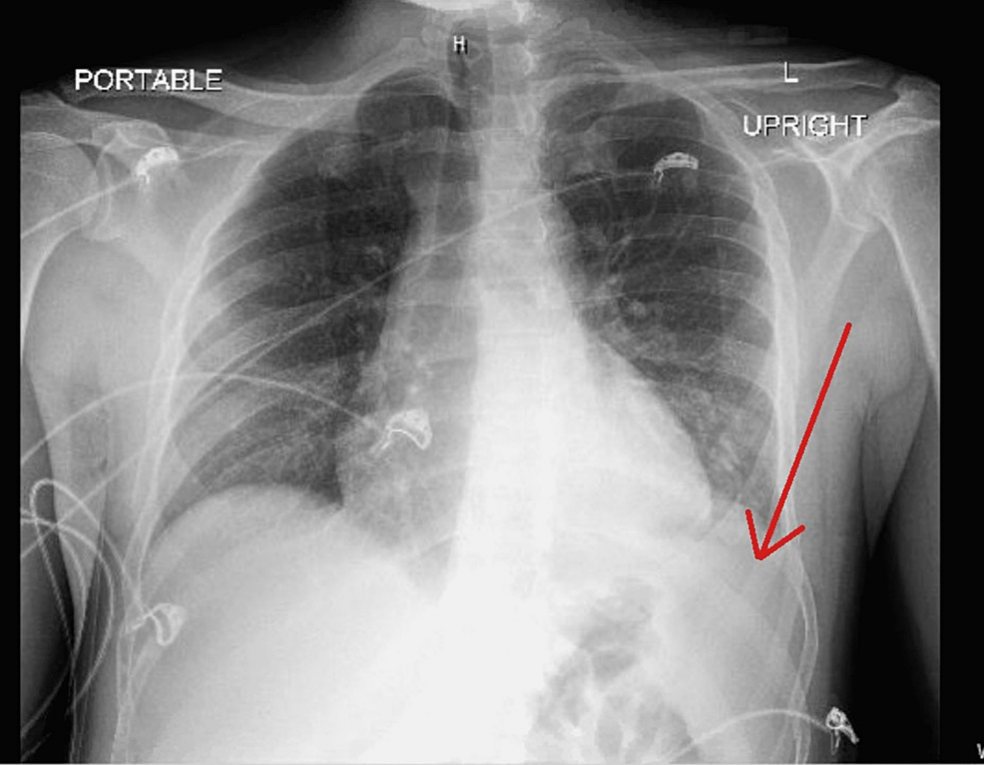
AP chest X-ray on January 2022. Red arrow points to small left pleural effusion.
